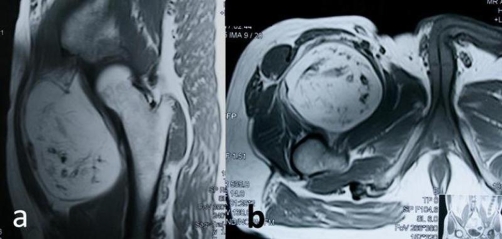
Magnetic Resonance Imaging revealed a well-circumscribed oval, lobular lipomatous mass that had high signal intensity on Tl-weighted images of the lesion. (a) Coronal section, (b) axial section.
